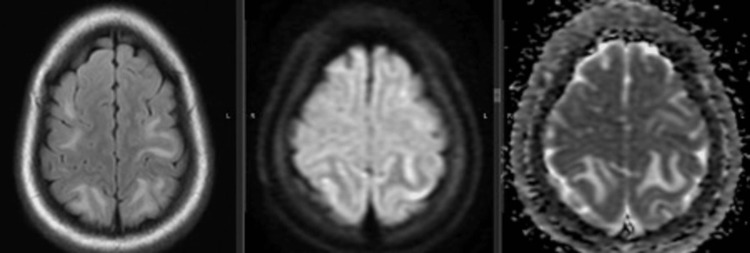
MRI brain axial section showing FLAIR (B) hyper-intensities in the parieto-occipital region extending to frontal lobes, without corresponding diffusion restriction (B & C), suggestive of vasogenic edema in a patient with eclampsia. MRI was suggestive of PRES. FLAIR, fluid-attenuated inversion recovery; PRES, posterior reversible encephalopathy syndrome
